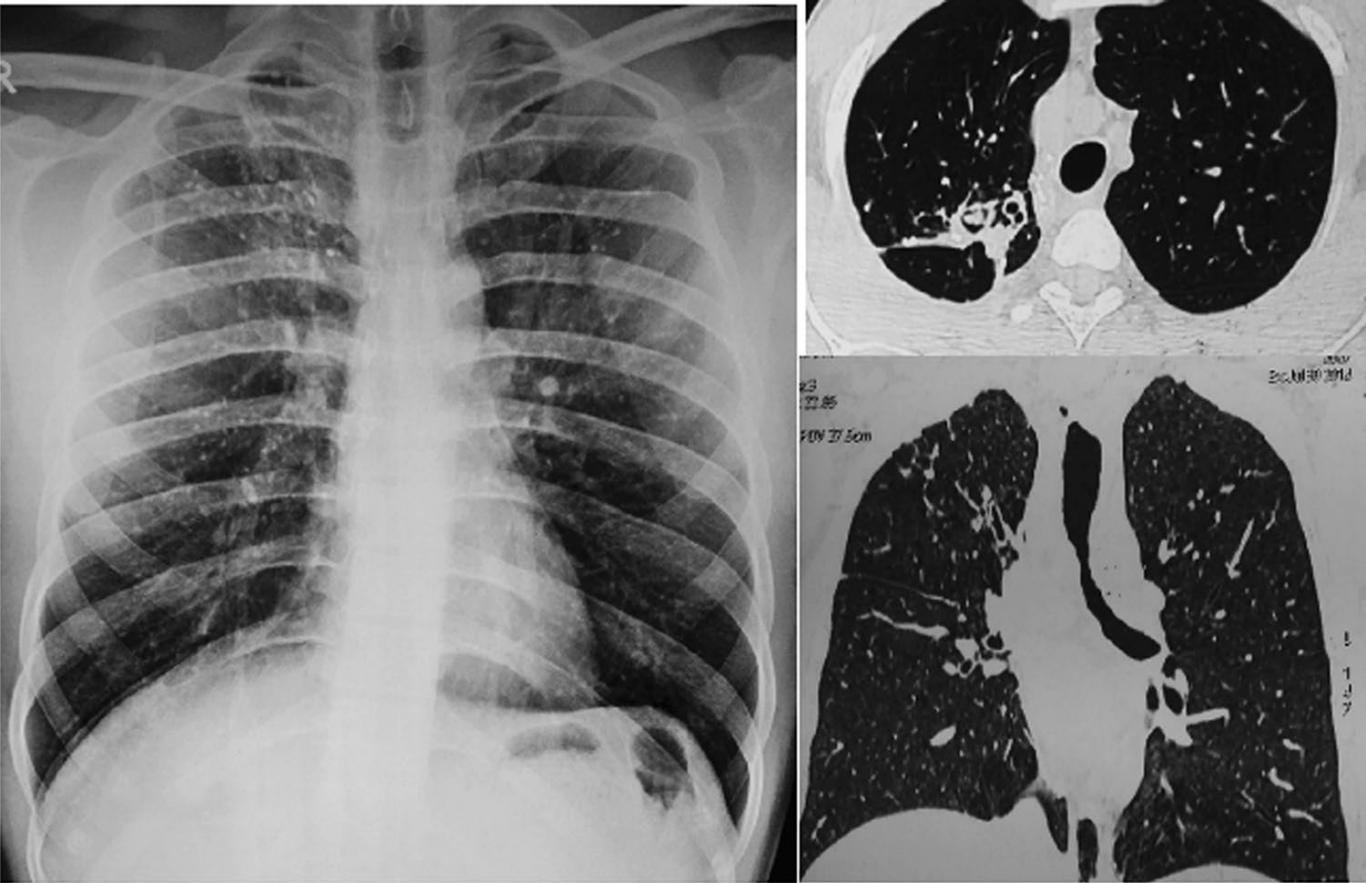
CXR showing *right upper* lobe opacity which on HRCT showed central bronchiectasis in *right upper* lobe anterior segment
